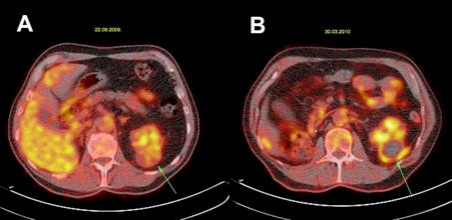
Patient number 2, an example of ^18^F-FDG-distribution in a renal mass before (A) and after 5 months cryoablation (B) at PET-CT. The light active hypointense uptake in the tumor in the left kidney before cryoablation (green arrow panel A) has changed to a hypometabolic area after ablation (green arrow panel B). The activity around the ablated tumor in the perirenal fat is possibly due to inflammatory tissue reaction. A contrast-enhanced CT scan as a control showed no enhancement of the ablation zone after treatment.
